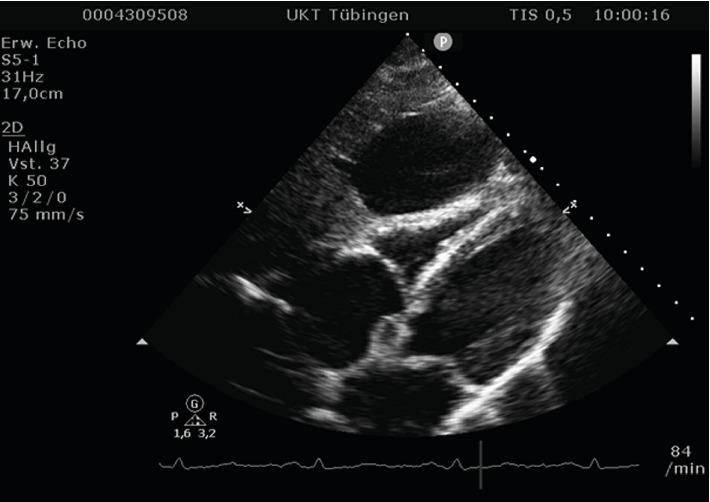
Echocardiogram, subxiphoidal view: cystic lesion of the liver compressing the right ventricle.
